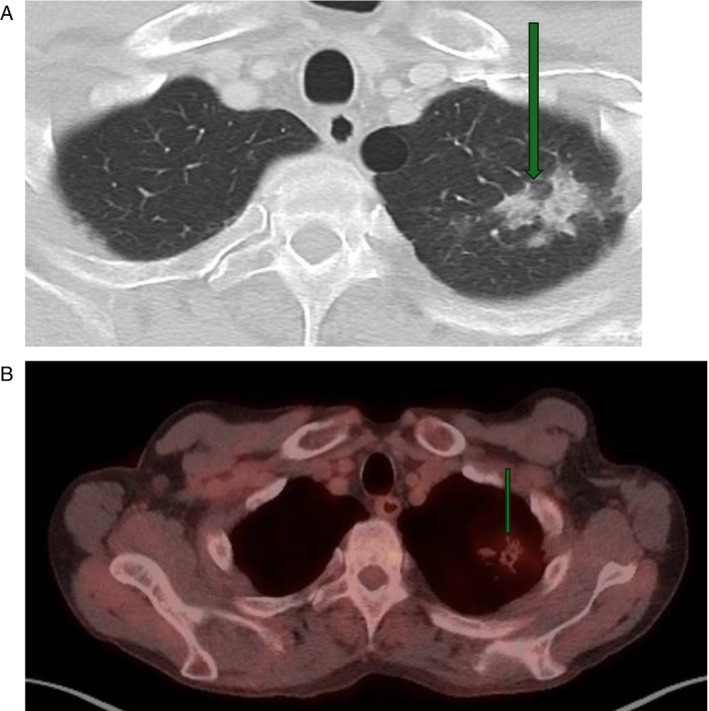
Previous CT chest showing patients malignancy (green arrow).
